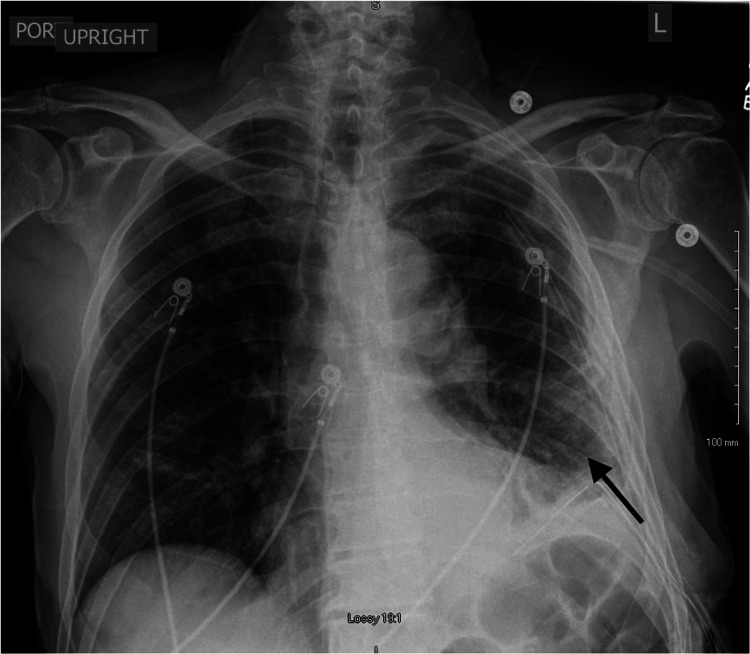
Initial Chest X-Ray An upright posterior-anterior chest x-ray showing left-sided lower lung opacity with obliterated costophrenic angle suggestive of pleural effusion (arrow).
